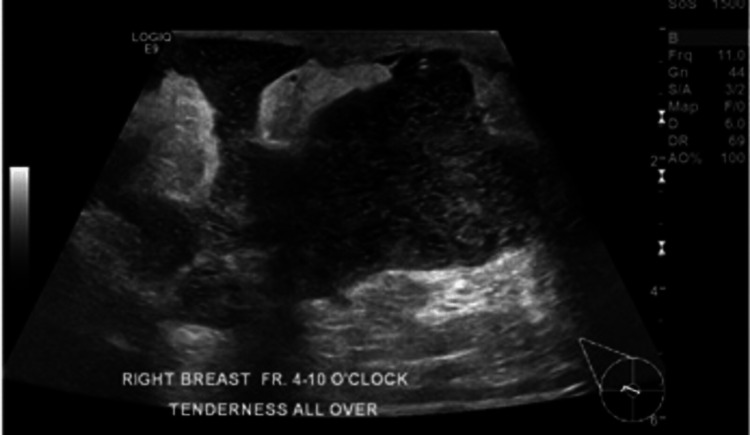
Right breast ultrasound showed new fluid collections at the retroareolar area of the right breast from 10:00 to 4:00.
